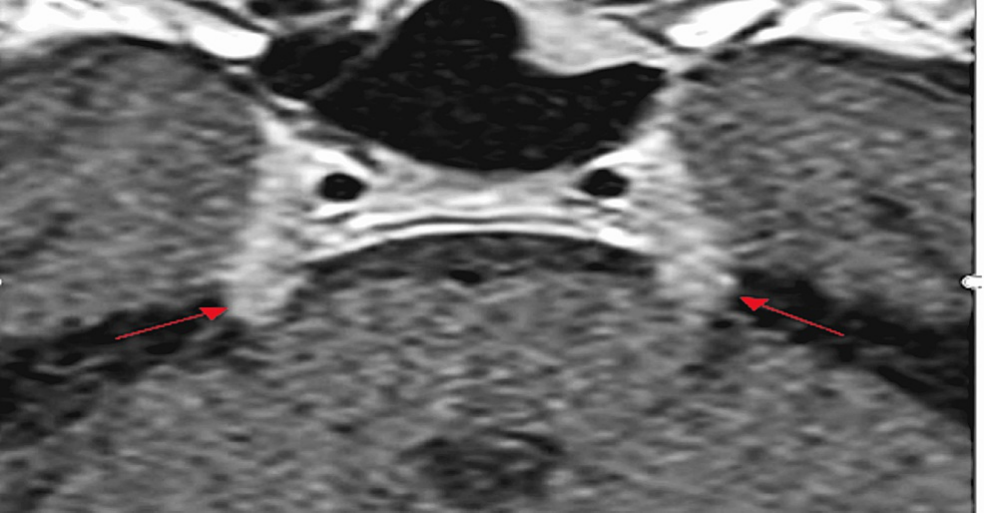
Bilateral thickened cranial nerve V (red arrows). MRI of the patient courtesy of Dr. Marc Swerdloff, August 2021.
